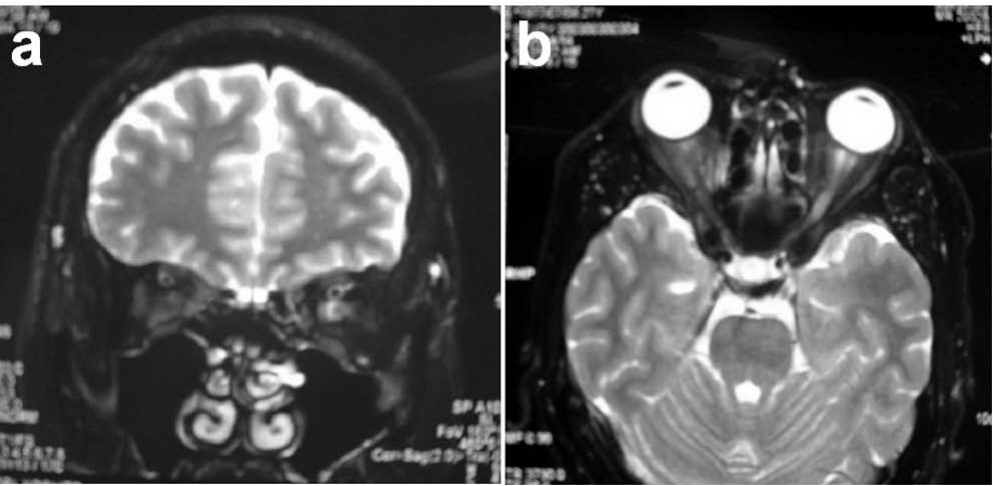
Magnetic resonance images of Case 3. T2-weighted coronal (a) and axial (b) images demonstrate strong hyperintensity around the left optic nerve of a 27-year-old Japanese man.
